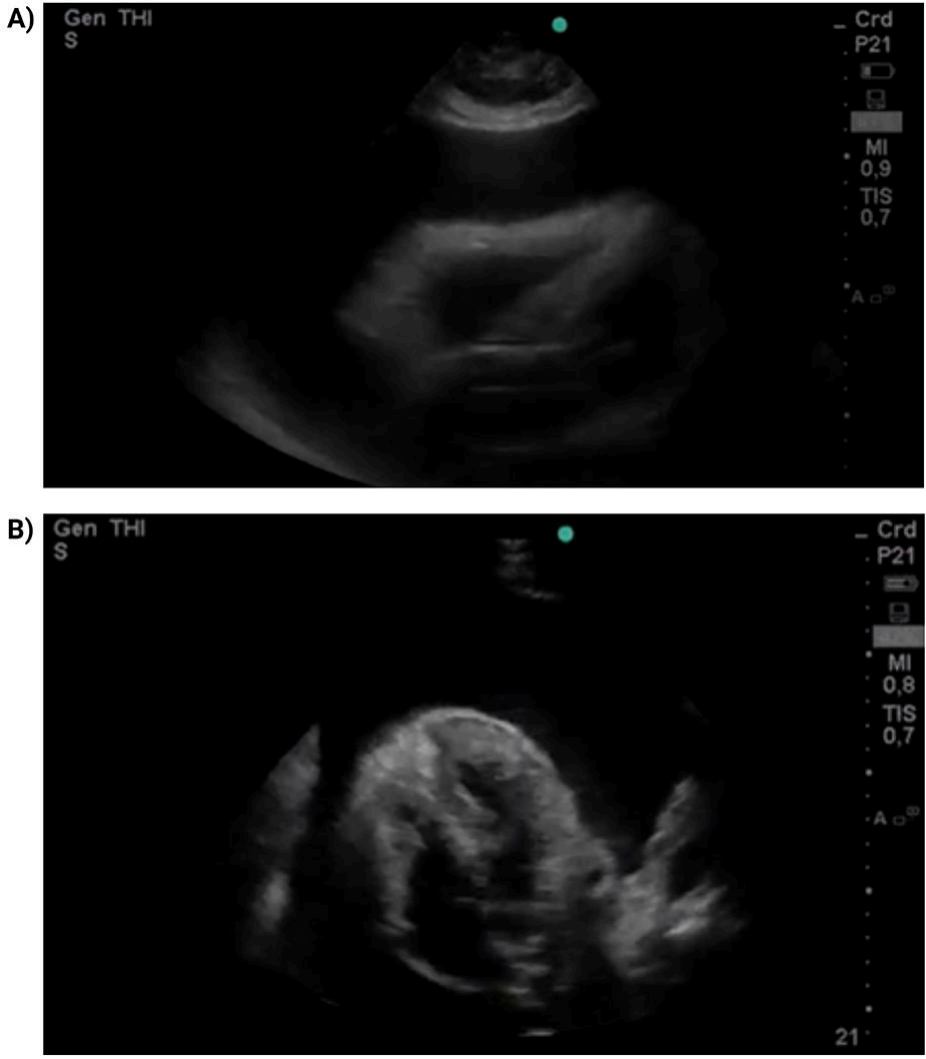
FoCUS parasternal long-axis view (A) and subxiphoid four-chamber view (B) A massive circumferential pericardial effusion is observed (28 mm in diastole), with collapse of right-sided chambers.
